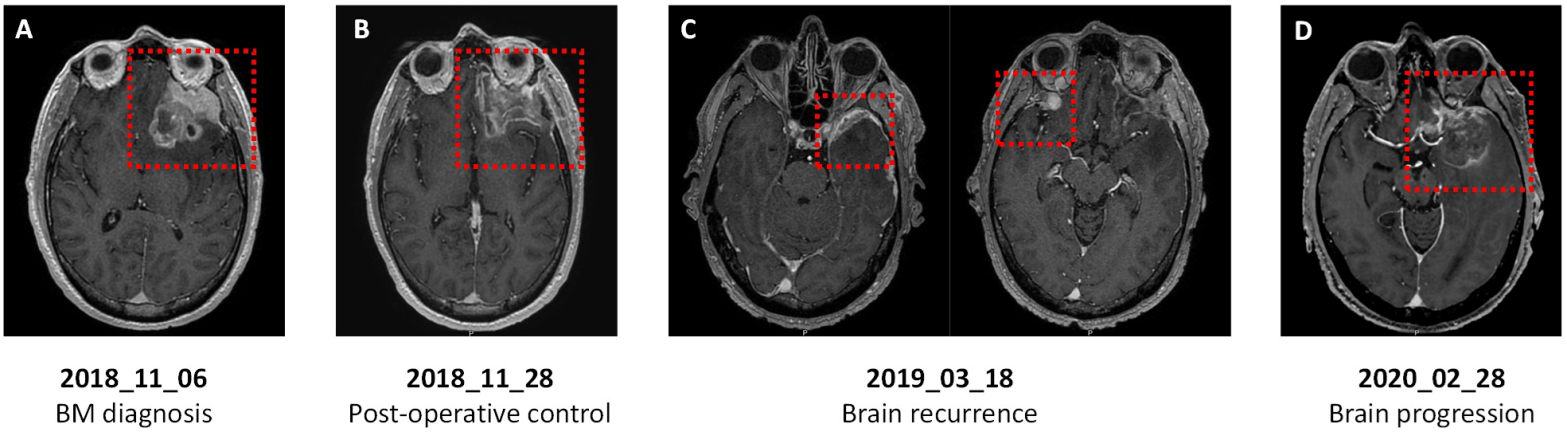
Axial T1-weighted brain magnetic resonance imaging (MRI) of a patient with PDAC and germline BRCA1 mutation. (A) At BM diagnosis: large left anterior frontal tumor in contact with the left optic nerve and perilesional edema. (B) After surgery: left fronto-orbital and dorsolateral ablation site without intraparenchymal enhancement. (C) At first brain recurrence: left frontal nodular meningeal tissue lesion (left) and new right inferior frontal enhanced nodular lesion with meningeal implantation (right). (D) At second brain recurrence: large left temporopolar and frontobasal lesion with significant perilesional edema.
